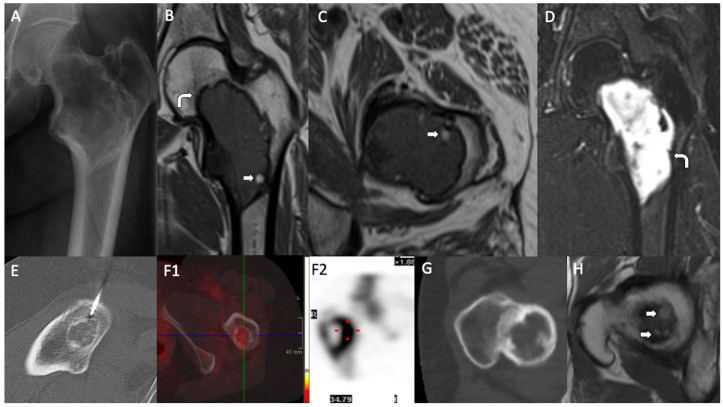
Radiological findings. LSMFTs of three patients. (A–D): (A) Anteroposterior radiography: geographic lucent lesion with a sclerotic margin and foci of matrix calcification, centered in the intertrochanteric region. (B) MIR, coronal and (C) MRI, axial T1WI: central medullary lesion that elicits low signal and peripheral hyperintense foci compatible with fat (white arrows). (D) MRI, coronal T2WI: high signal. Low-signal sclerotic rim, hypointense on T1WI and T2WI (curve arrows). (E,F): (E) Axial image, CT-guided biopsy: lytic lesion with internal calcified foci. (F1) (SPECT) and (F2) (bone scintigraphy): moderate and heterogeneous uptake of the lesion (red dot: intense uptake area). (G,H): (G) Axial CT: lytic lesion with a sclerotic rim. (H) MRI, axial T1WI: small foci of T1 high signal at the superior aspect of the lesion, suggestive of fat (white arrows).
